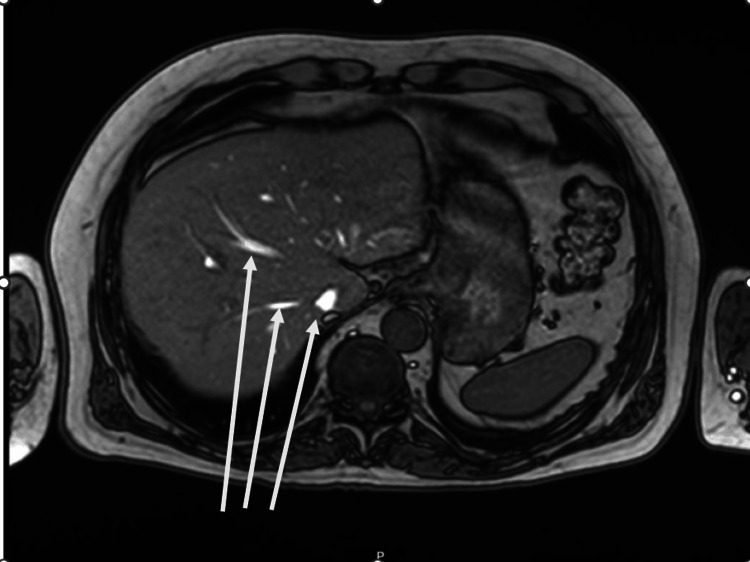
MRI with signs of cholangitis and resolving abcess
